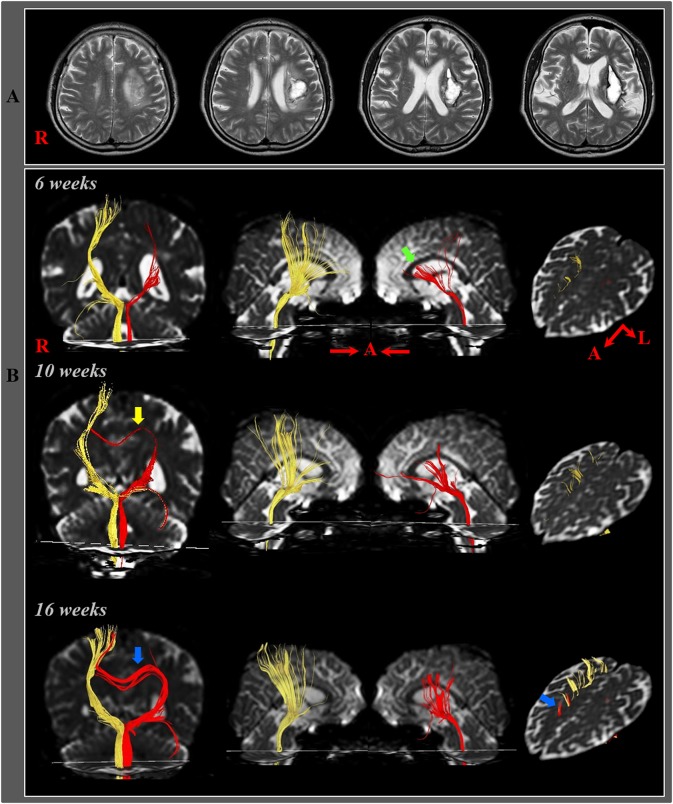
(A) T2-weighted MR images showed a hematoma in the left corona radiata and basal ganglia at 6-weeks after onset. (B) Results of diffusion tensor tractography (DTT). On 6-, 10-, and 16-week DTTs, the CRPs in the right hemispheres originated from the premotor cortex and primary motor cortex and descended through the known CRP pathway. By contrast, in the left (affected) hemisphere, the CRP showed severe narrowing with discontinuation of the anterior fibers (green arrow) on 6-week DTT, however, the discontinued fibers of the CRP were connected to the corpus callosum through the transcallosal fibers (yellow arrow) on 10-week DTT and became thicker and connected to the right cerebral cortex *via* the corpus callosum (blue arrow) on 16-week DTT [Reprinted with permission from Jang and Yeo (23)].
